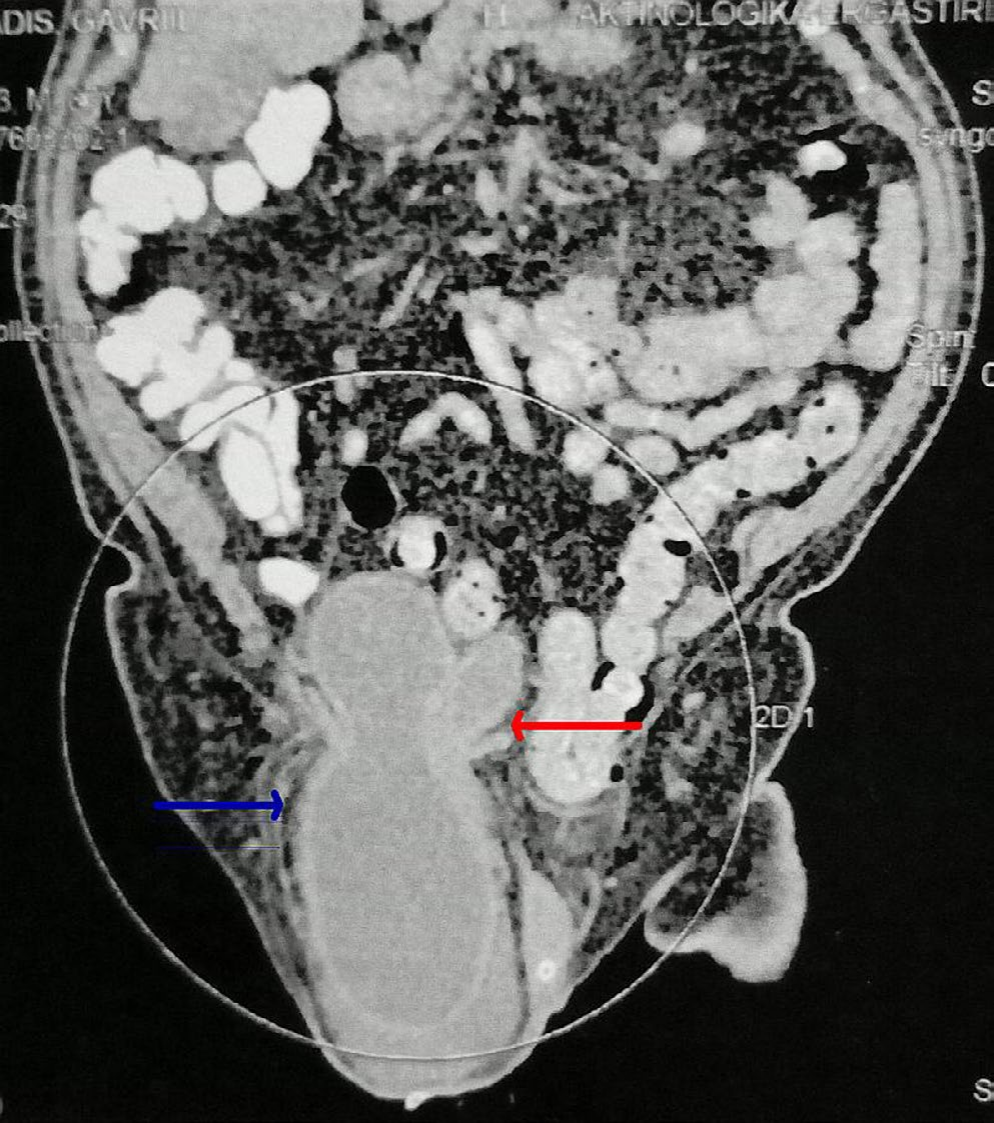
CT showed the bladder apex, body, and fundus (blue arrow) along with the trigone (red arrow) protruding into the hernia sac without herniation of bowel loops
